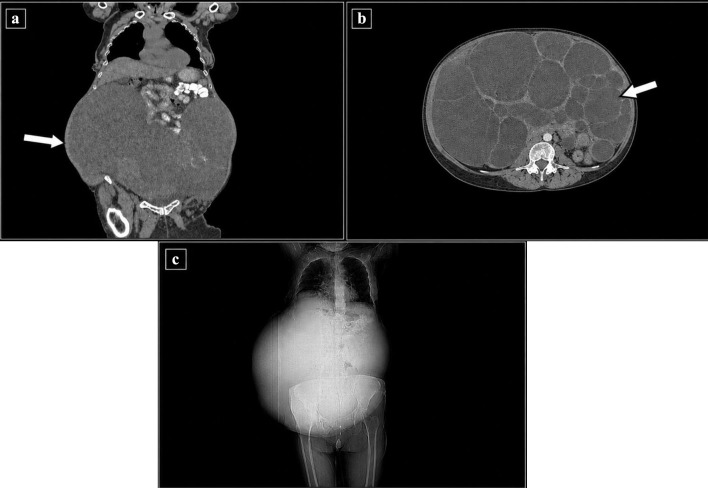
Computed tomography (CT) scan examination revealed large intra-abdominal tumor. (a) Coronal contrast-enhanced CT image showing a large intra-abdominal tumor occupying the lower abdomen. Arrow indicates giant tumor. (b) Axial contrast-enhanced CT image demonstrating the multi-cystic nature of the tumor. Arrow indicates cysts. (c) CT scout image demonstrating the marked abdominal distention caused by the large intra-abdominal mass.
